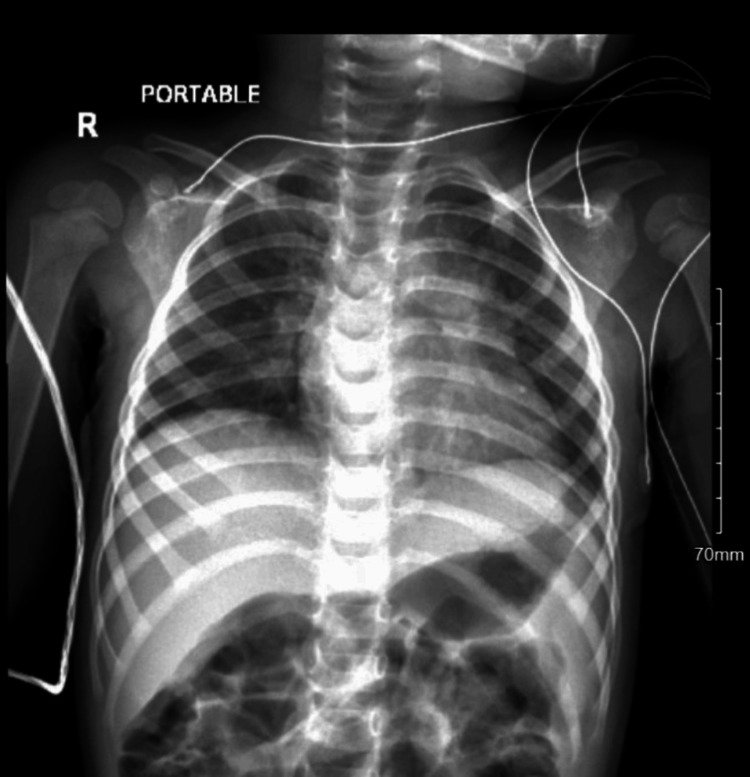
Postoperative chest X-ray showing complete removal of the foreign body with clear esophageal passage and no residual radiopaque density, verifying successful intervention.
